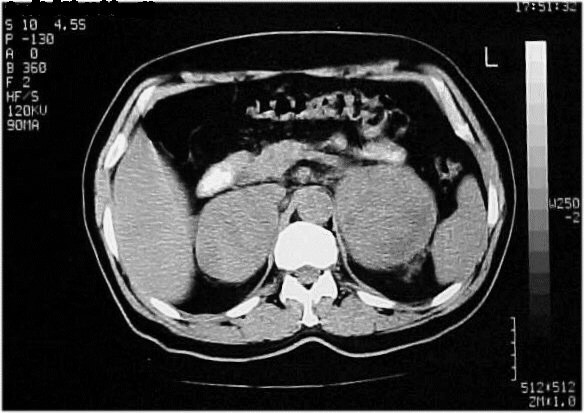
CT-scan of the abdomen showing large masses (98 × 85 mm right and 90 × 65 mm left) of heterogeneous texture, with hemorrhagic and necrotic elements within both adrenal glands.
